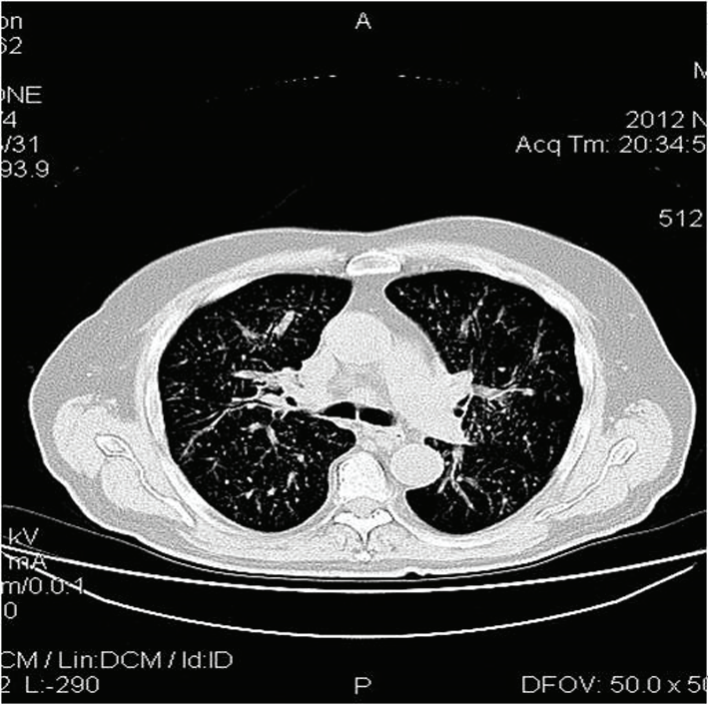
Chest CT shows multiple diffuse smooth thickening of the interstitial pulmonary parenchyma and an expanded right paratracheal lymph node with a diameter of 2.5 cm.
